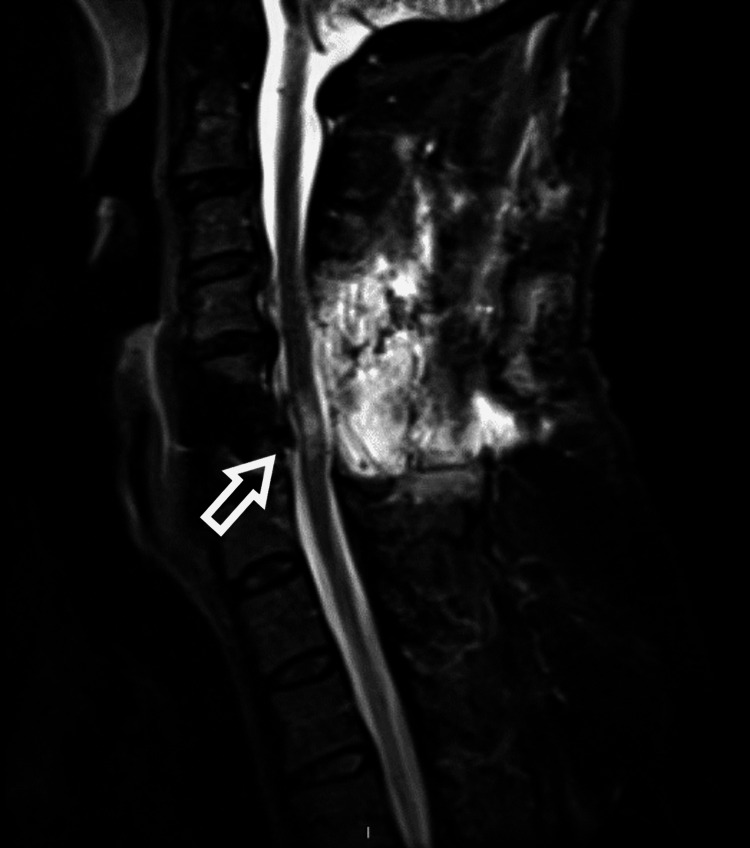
Magnetic resonance imaging scan sagittal view, post posterior laminectomy showed signal changes at C5/6. Magnetic resonance imaging scan revealed the high signal changes at the level C5/6 (at level of cord swelling) after the second surgery. The posterior spinal decompression provides the spinal cord enough space to demonstrate signal changes from previous surgery.
